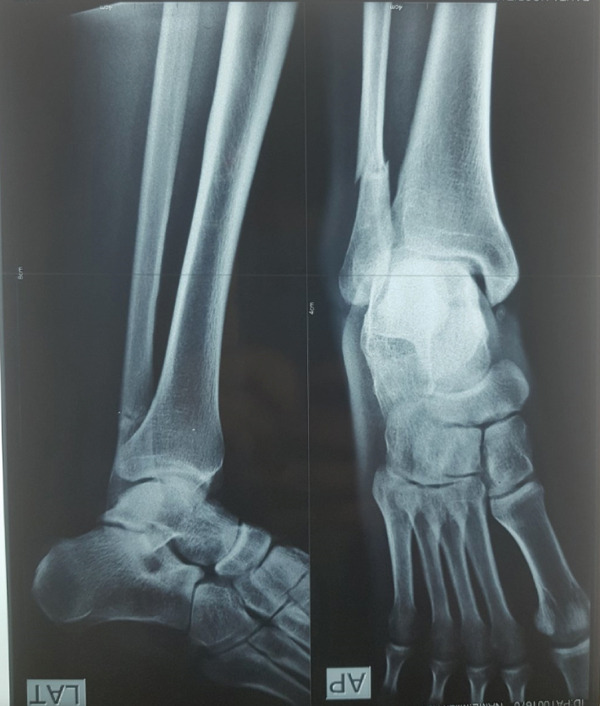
Anteroposterior and lateral plain radiographs showing transverse fracture of the right distal fibula around 5 cm proximal to the lateral malleolus with minimal displacement.
